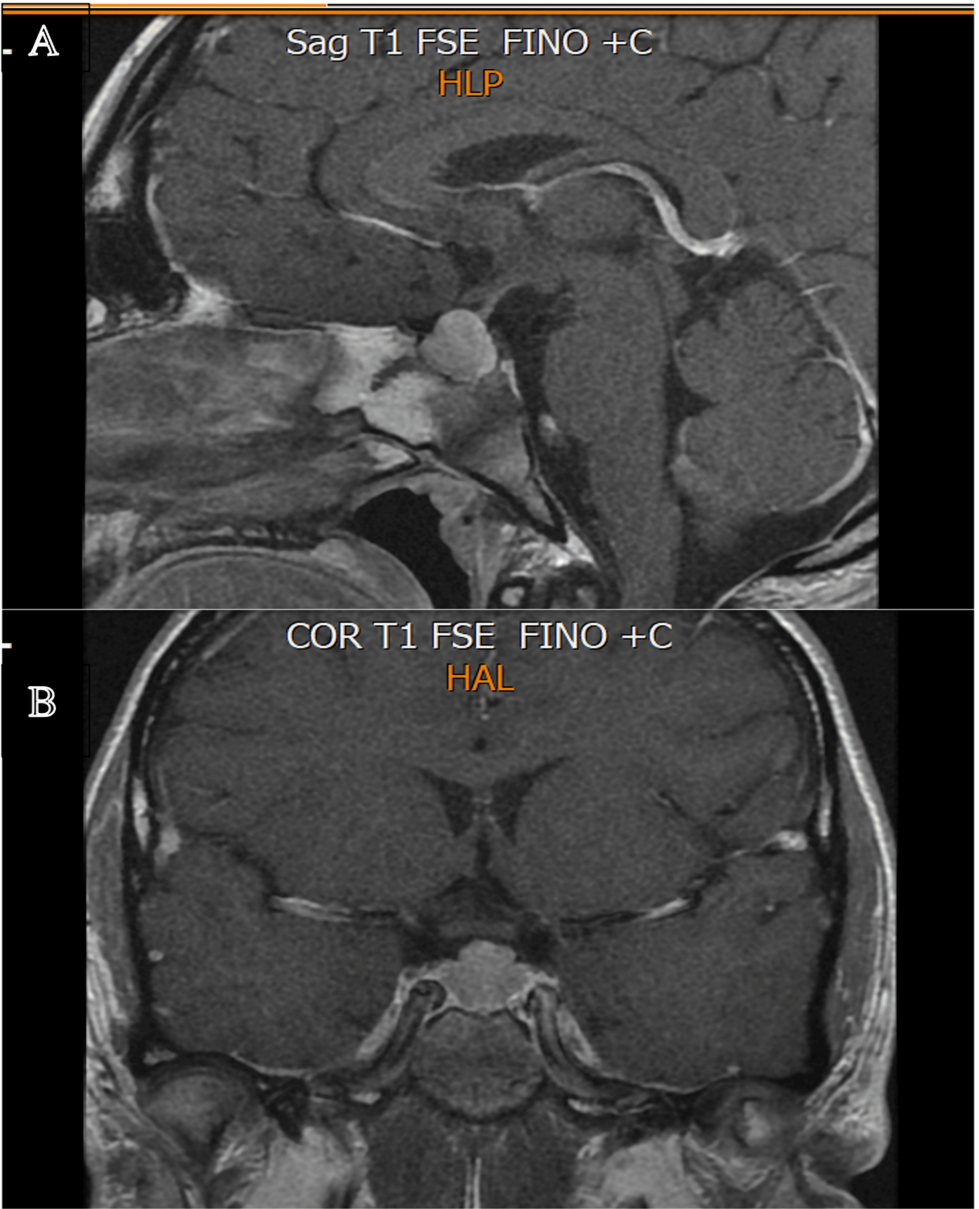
MRI images showing pituitary enlargement, an expansive intrasellar and suprasellar lesion measuring 14×13×13 mm, were initially interpreted as a pituitary adenoma. (A) sagittal view; (B) coronal view
